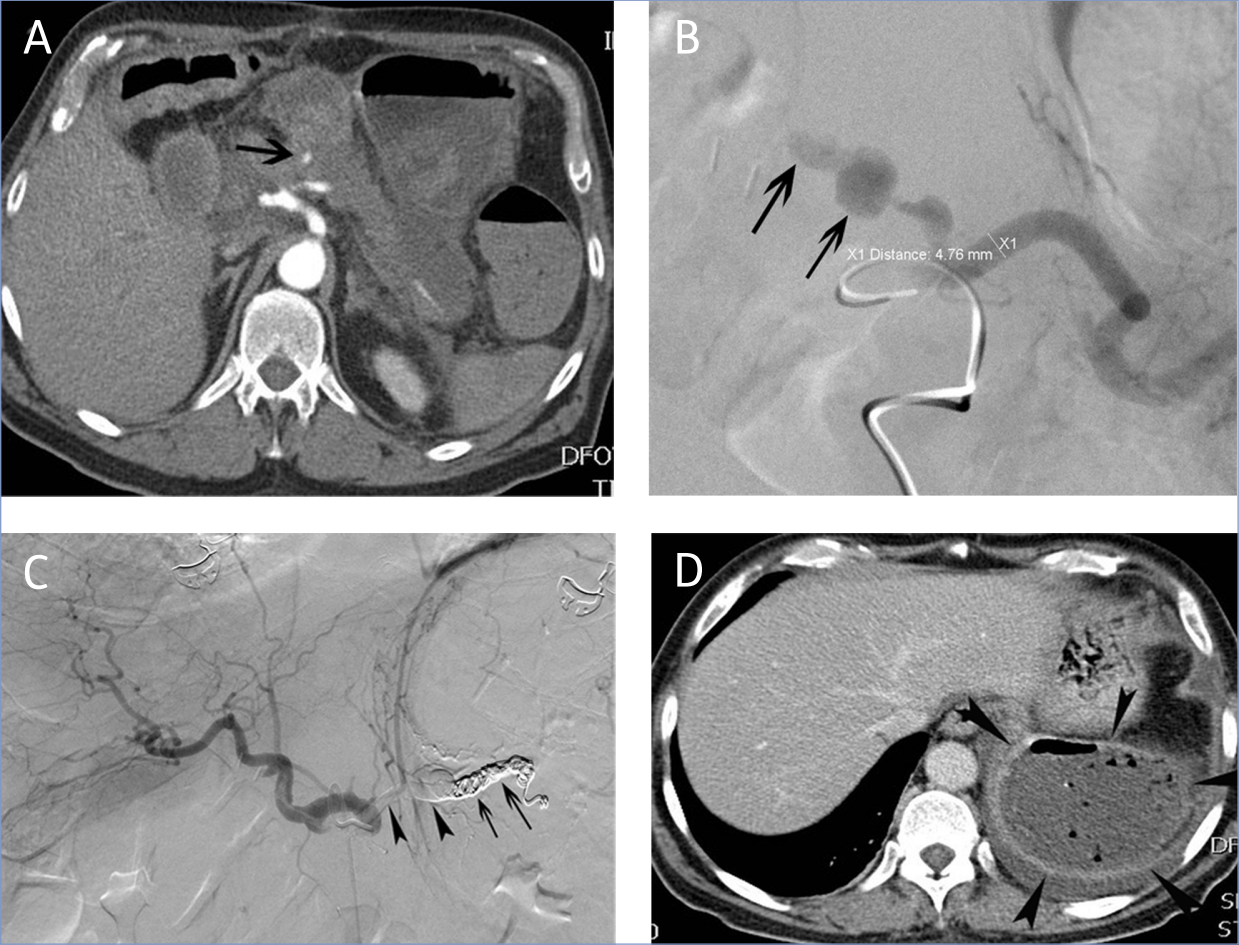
Main splenic artery embolization for postoperative hemorrhage. (A) CT-angiography in a 64-year-old man 19 days after pancreatico-duodenectomy revealed contrast extravasation (arrow) in the proximal part of the main splenic artery. (B) Corresponding selective splenic arteriography confirmed the contrast extravasation and a pseudoaneurysm (arrows). (C) Embolization of the hemorrhage was performed with a combination of coils (arrows) and glue (arrowheads). (D) CT eight days after embolization revealed multiple air bubbles in a completely necrotic splenic tissue compatible with abscess.
